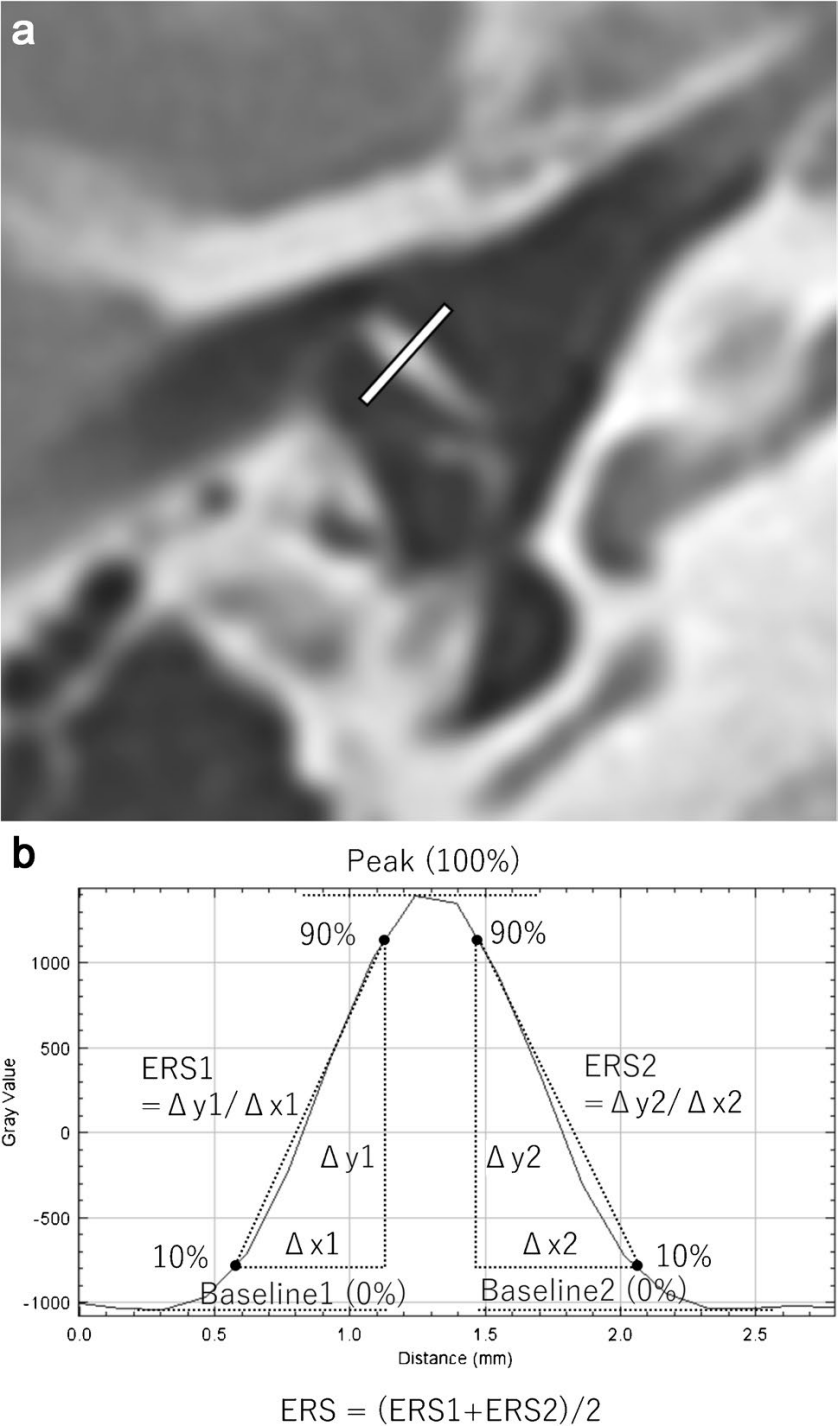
A A linear region of interest was placed passing perpendicular to the handle of the malleus on axial image. b Based on the CT attenuation profile along the region of interest, the edge rise slope (ERS), which was averaged for both slopes, was calculated
